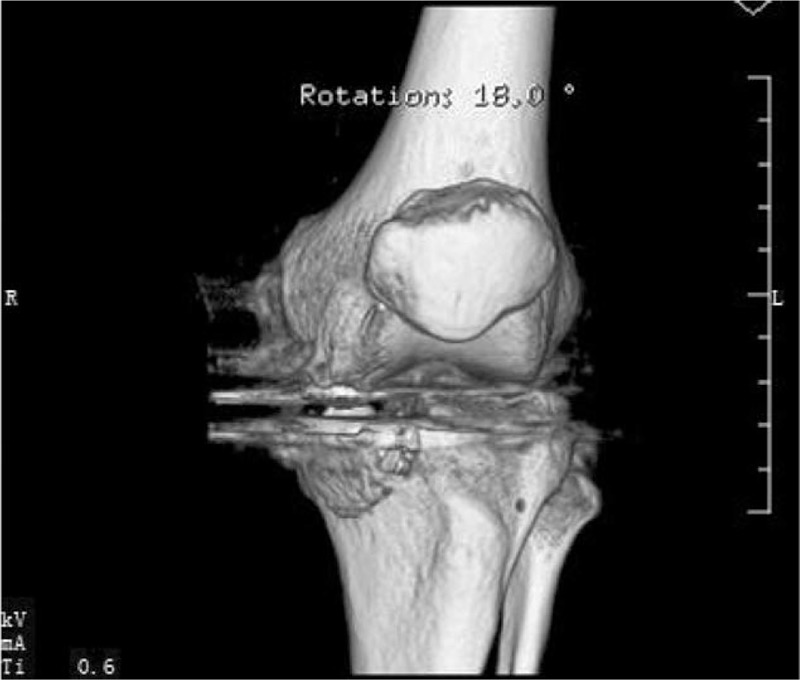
CT showing medial malleolus fracture of the left knee. CT = computed tomography.
